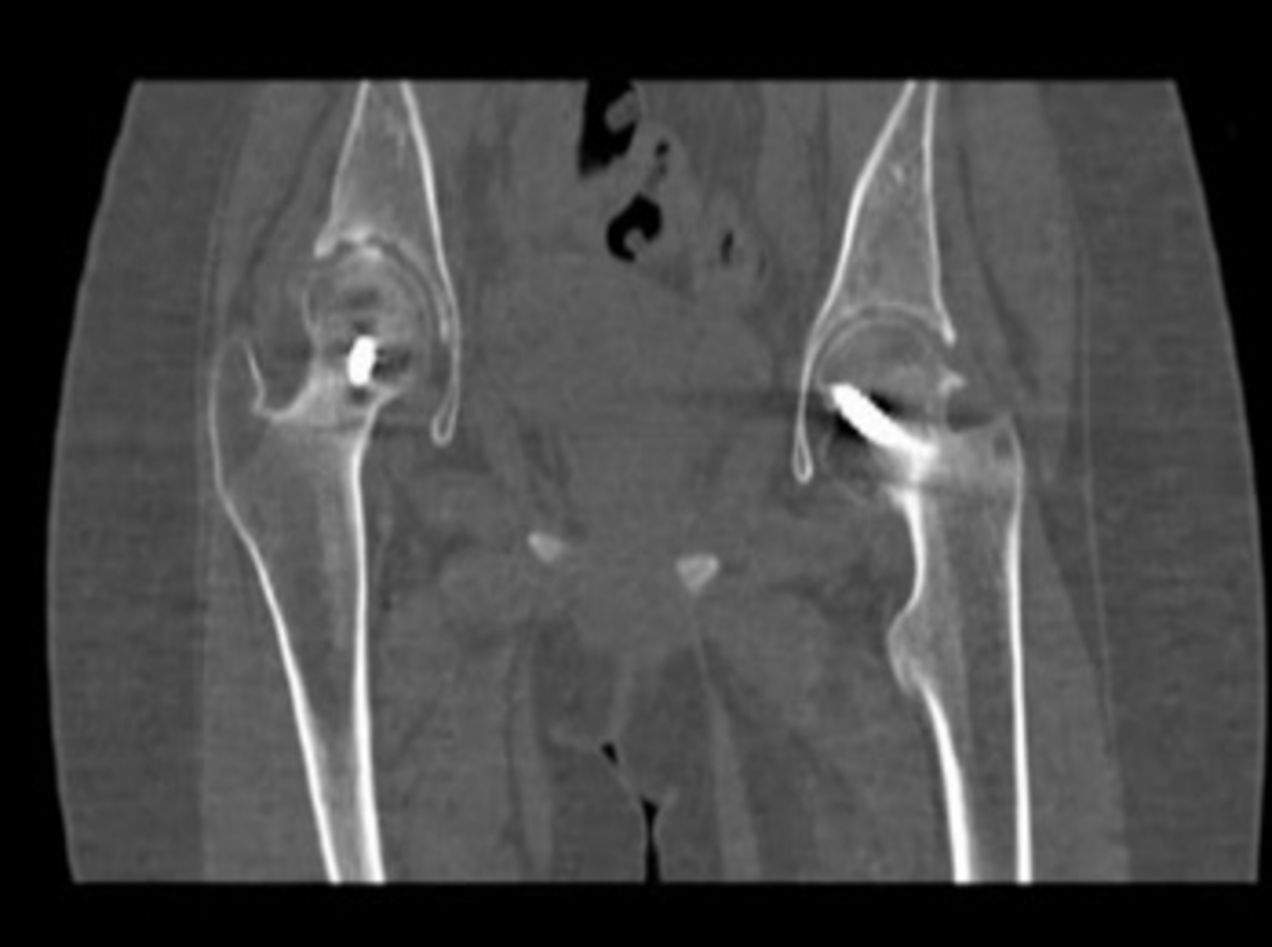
One-year postoperative coronal CT scan The left hip showed subchondral sclerosis with lateral osteophytes. On the right side, the fixating screw was noted to penetrate into the articular surface of the femoral head with some osteoarthritic changes. CT: computed tomography CT: computed tomography
